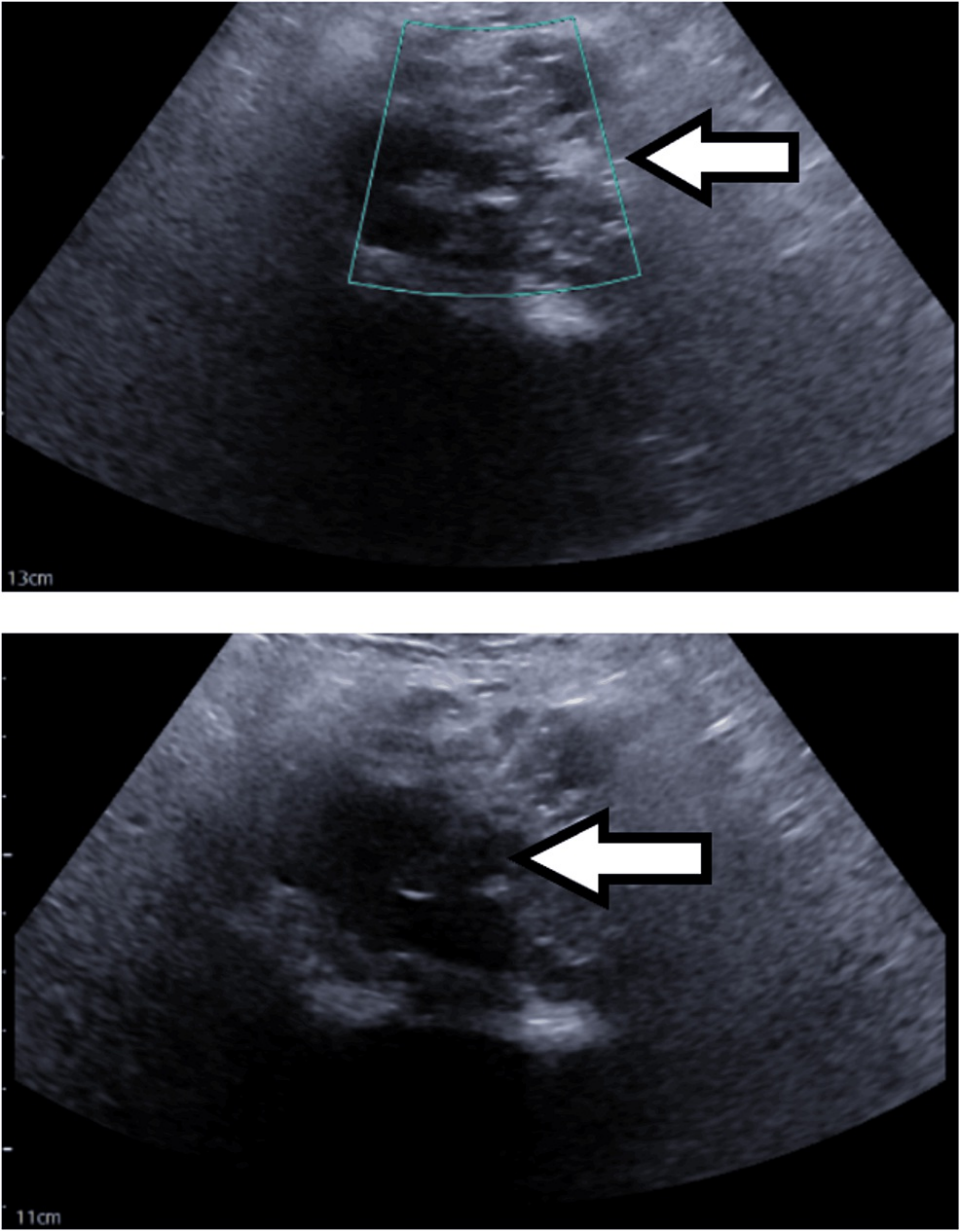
Ultrasound-guided percutaneous right iliac soft tissue mass biopsy
